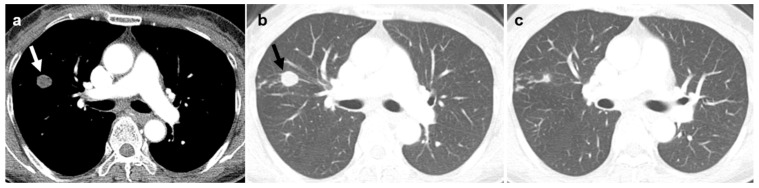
A female patient with nontuberculous mycobacterial infection. (a,b) Chest CT reveals a 1.6 cm round nodule (arrow) with internal low attenuation in the right upper lobe. Percutaneous core needle lung biopsy indicated chronic inflammation with extensive necrosis, and tissue PCR results were positive. (c) Adjacent associated small nodular infiltrations are present in the right upper lobe.
